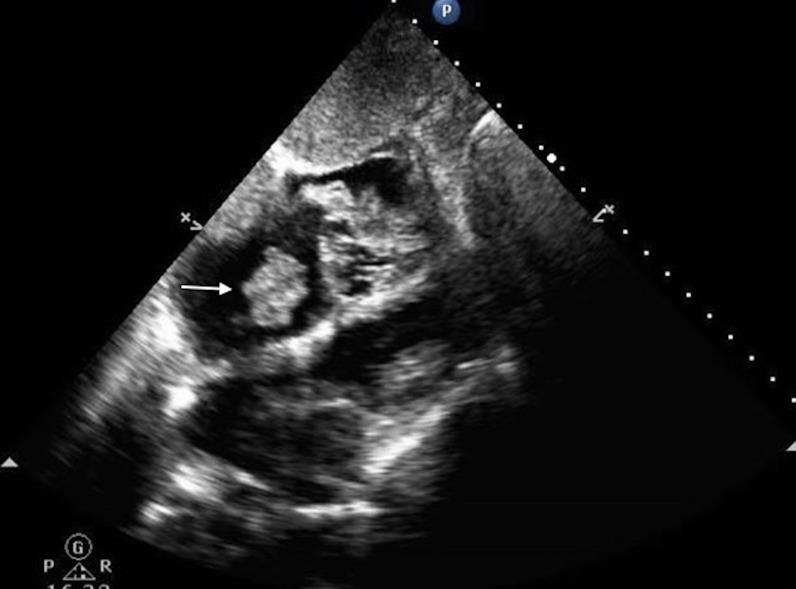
The image from the echocardiogram demonstrating a 2.1 x 2.2 cm thrombus in the right atrium.
